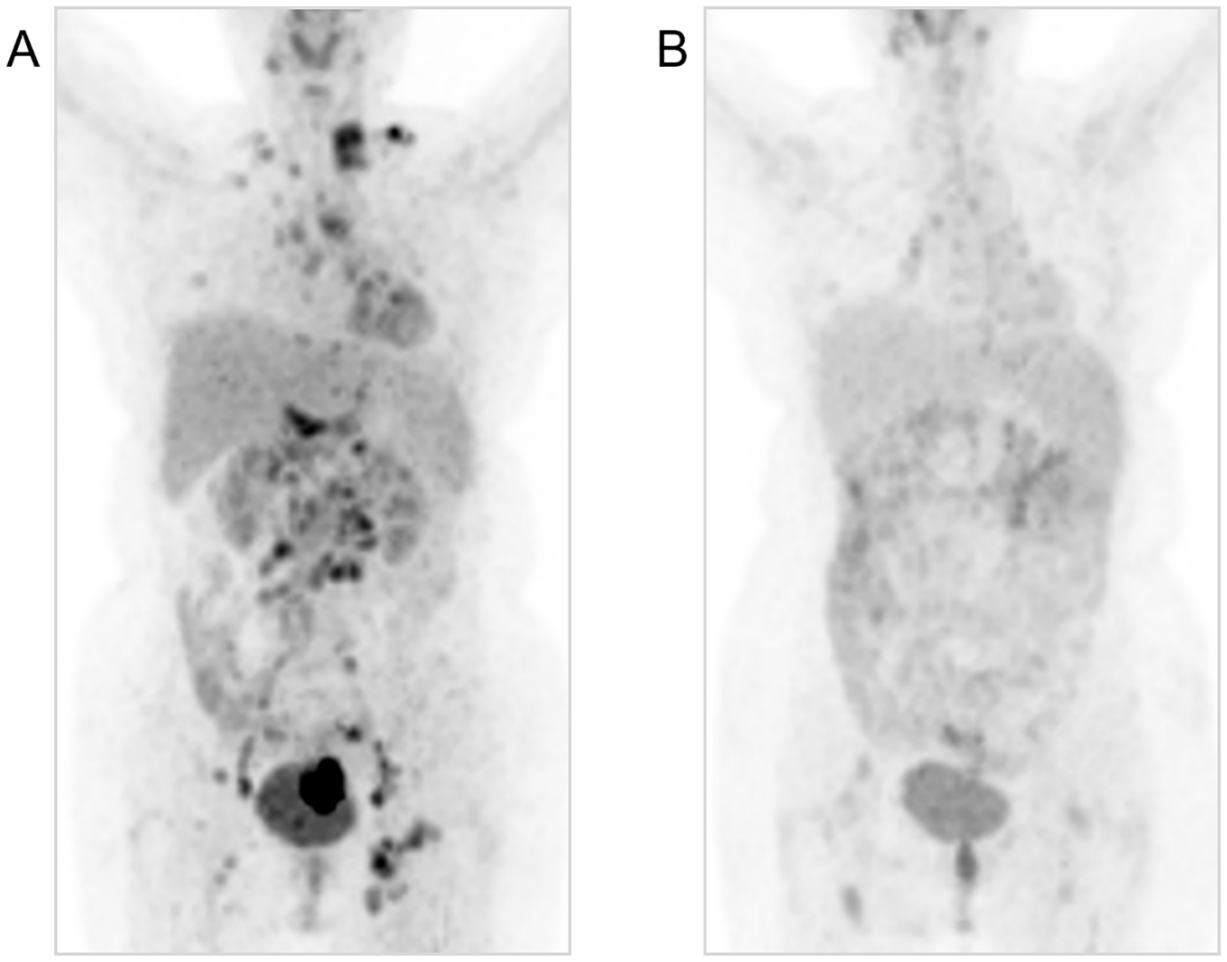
Comparison of PET-CT before traetment (A) and after treatment for 1 years (B).
